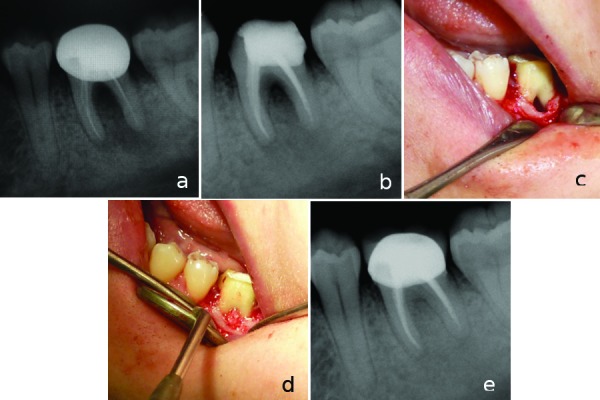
a) Initial radiograph of tooth #36. b) 3 months recall radiograph showing bone lesion in the furcation area. c) Intraoral film showing furcation lesion during periodontal surgery. d) Intraoral film showing bone graft material placement to the furcation lesion. e) Two year recall radiograph showing complete healing of the furcation lesion.
